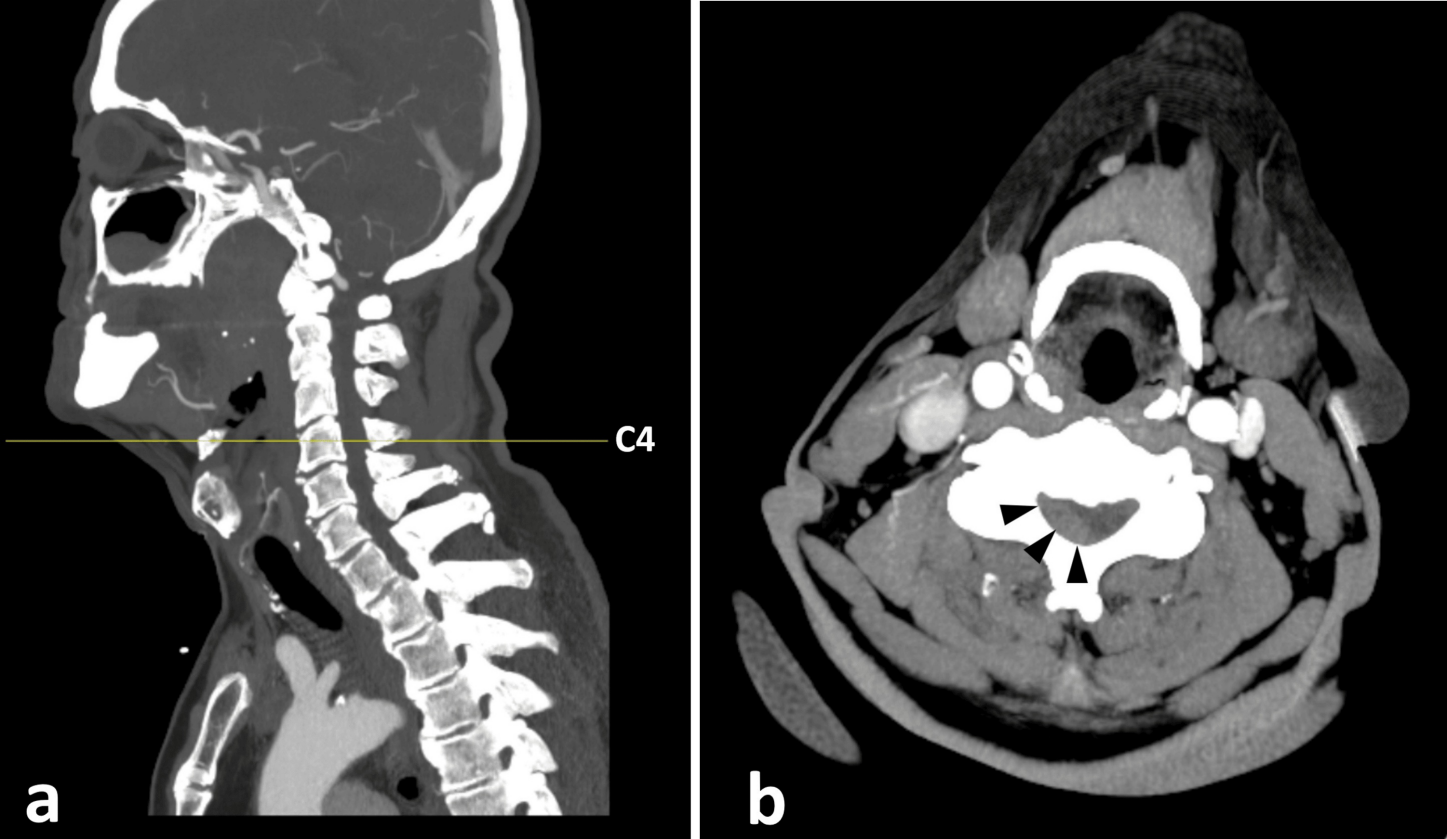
CT angiogram from the arch of the aorta to the circle of Willis showed a mildly hyperdense and mildly enhancing epidural lesion (black arrows) centered at C4 (extending from C3 to C5) with compression and contralateral displacement of the cervical cord suggestive of a mass lesion or an epidural hematoma. (a) Sagittal view with yellow line indicating cord level of image (b). (b) Axial view at the level indicated on image (a) CT: computed tomography
